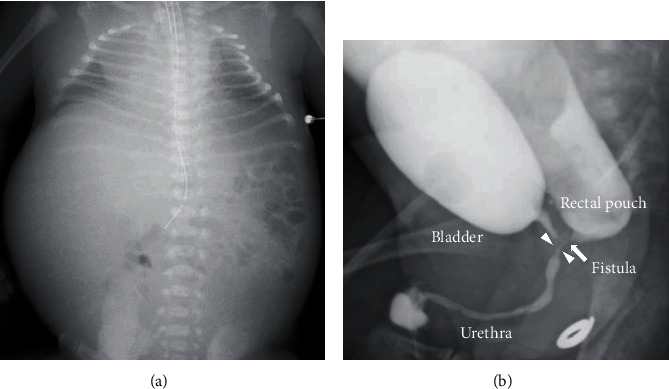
Neonatal chest X-ray and cystourethrography: (a) chest X-ray on day 1 showing poor lung expansion and abdominal expansion; (b) cystourethrography showing a patent rectourethral prostatic fistula into the rectal pouch and a patent urethra. The arrowheads indicate urethral stenosis distal to rectourethral fistula. The arrow indicates rectourethral prostatic fistula.
